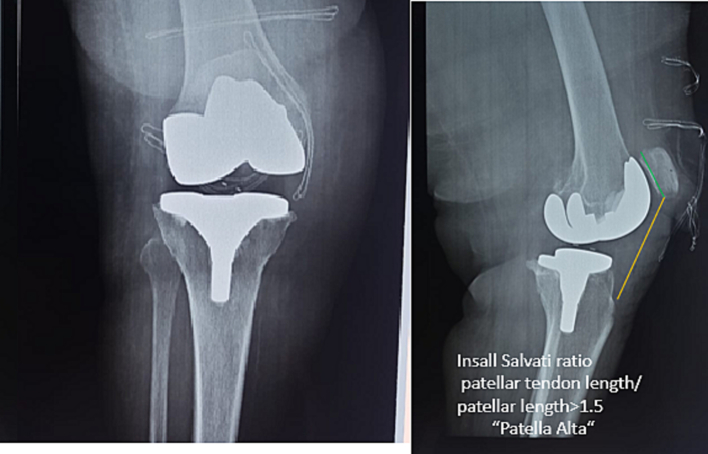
X-ray of the knee on admission: yawning on the medial side, deviation radiopaque markers of the polyethylene, and elevation of the patella in profile the Insall Salvati ratio is equal to 1.6 synonym of patella alta.
